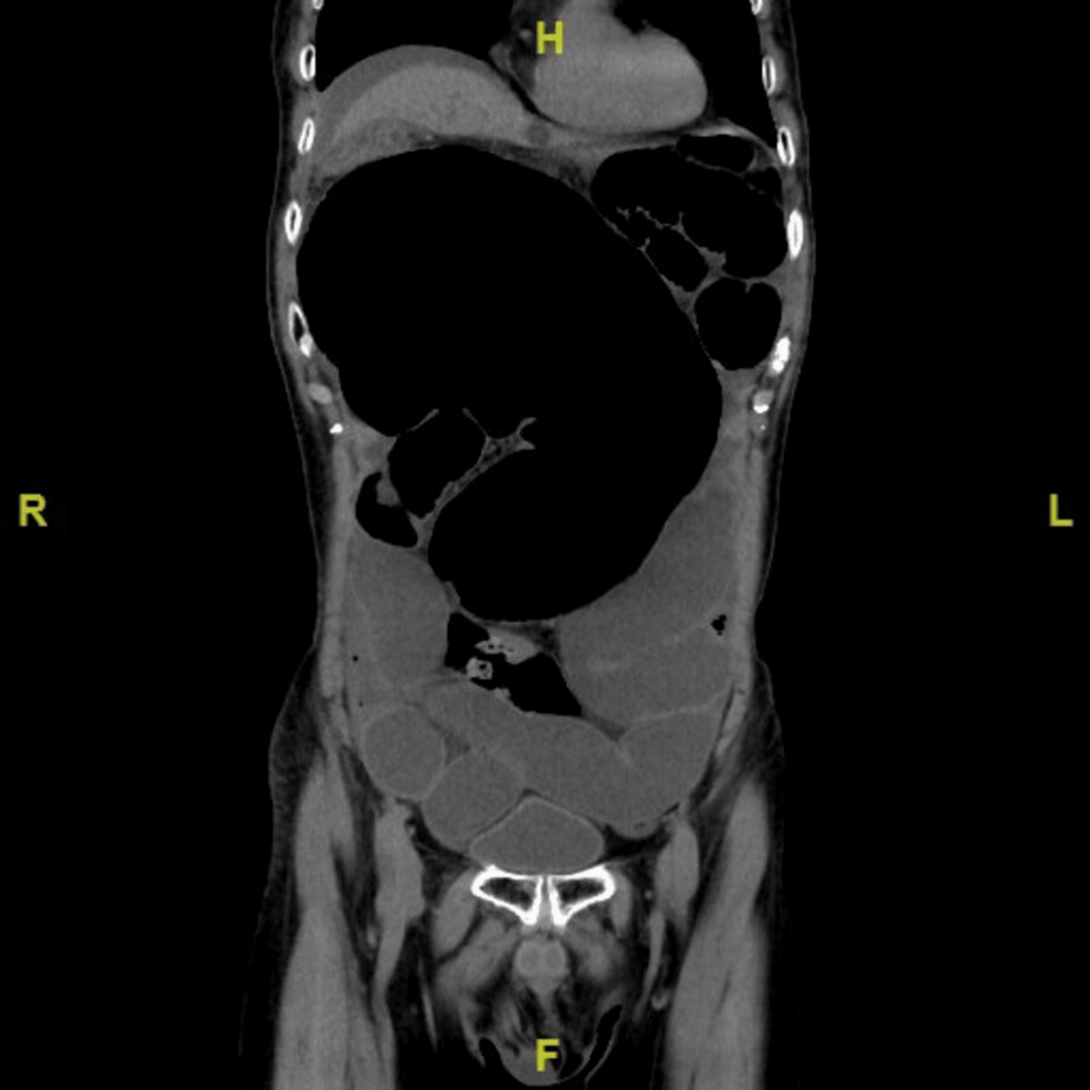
CT scan showed cecal dilation consistent with cecal volvulus Non-contrast CT on admission showing marked cecal dilatation consistent with cecal volvulus. No free air or signs of bowel necrosis are observed.
